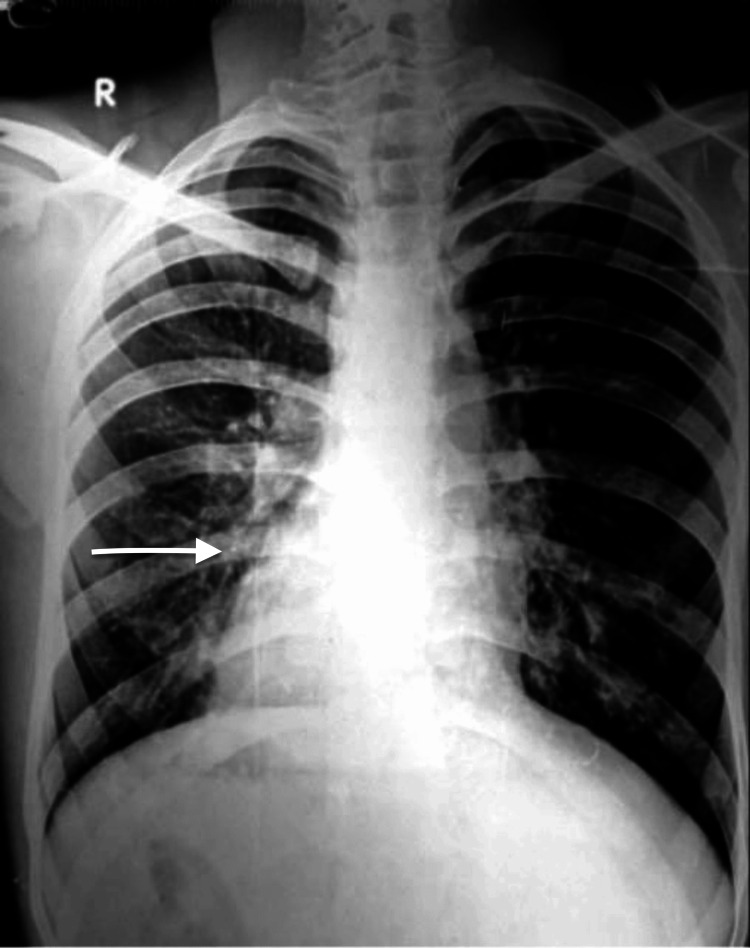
Chest X-ray revealing dextrocardia
